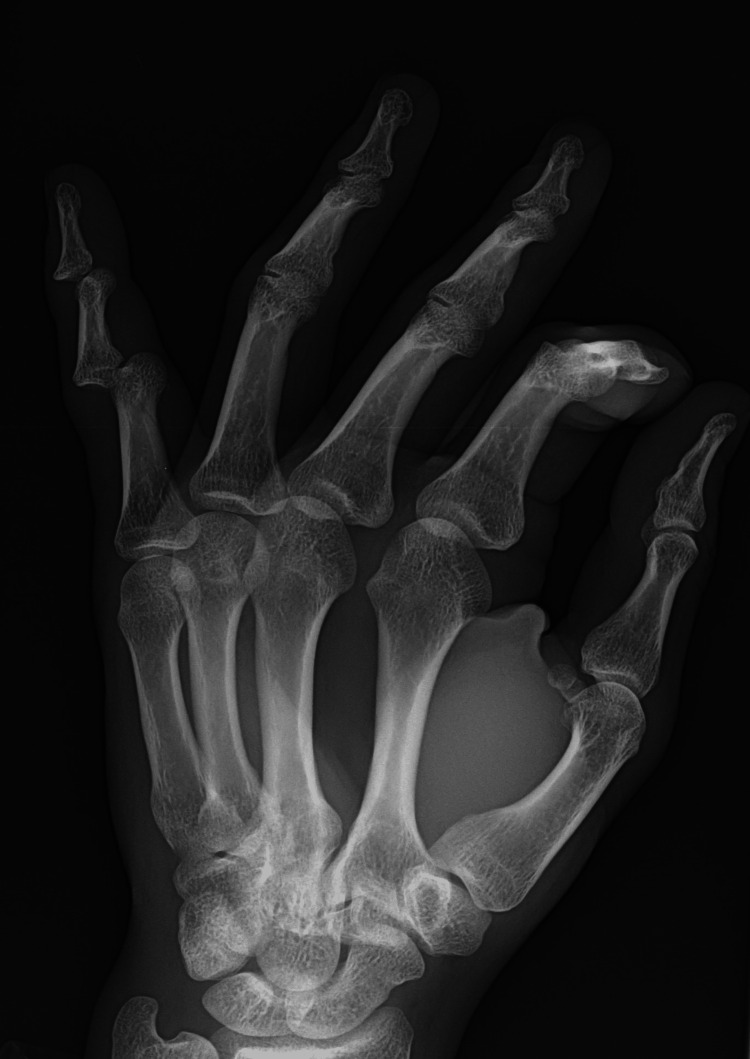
Oblique left-hand radiograph. Oblique radiograph of the patient’s left hand showing double dislocation of both proximal and distal interphalangeal joints of the little finger.
